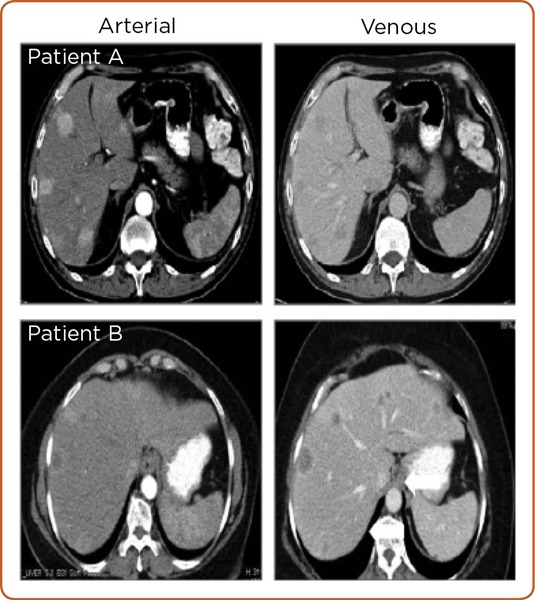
Liver CT. Images courtesy of MD Anderson Cancer Center.
